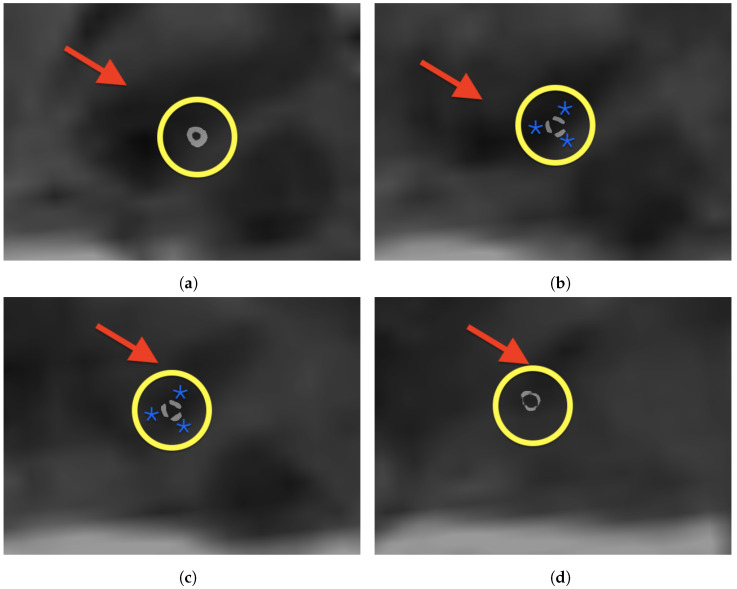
Axial non-contrast T2-weighted MRI with DynaCT superimposed using alpha blending. The subthalamic nucleus is displayed as a T2-hypointense streak (red arrow). All parts of the electrode that are relevant for optimizing the stimulation can be clearly delineated (yellow circle). The image sequence goes from caudal (a) to cranial (d). (a) Proximal circular contact within STN. (b) Proximal segmented contacts with blue stars indicating the segments for steering the electric field. (c) Distal segmented contact with blue stars indicating the segments for steering the electric field. (d) Distal circular contact.
